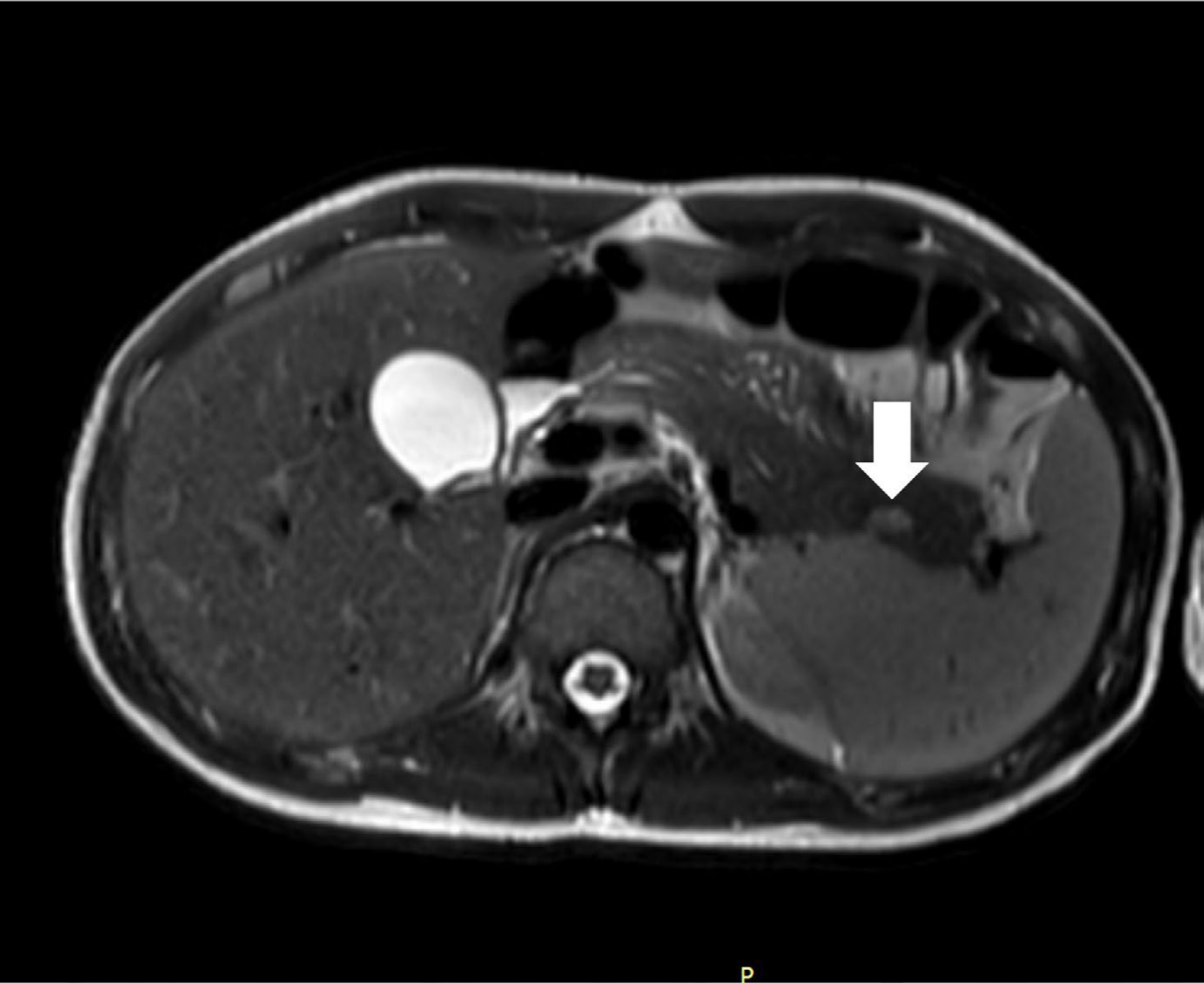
Abdominal magnetic resonance imaging. Axial T2‐SPAIR gradient‐echo MRI showing a hypervascular intrapancreatic nodule at the posteroinferior aspect of the pancreatic tail (white arrows).
